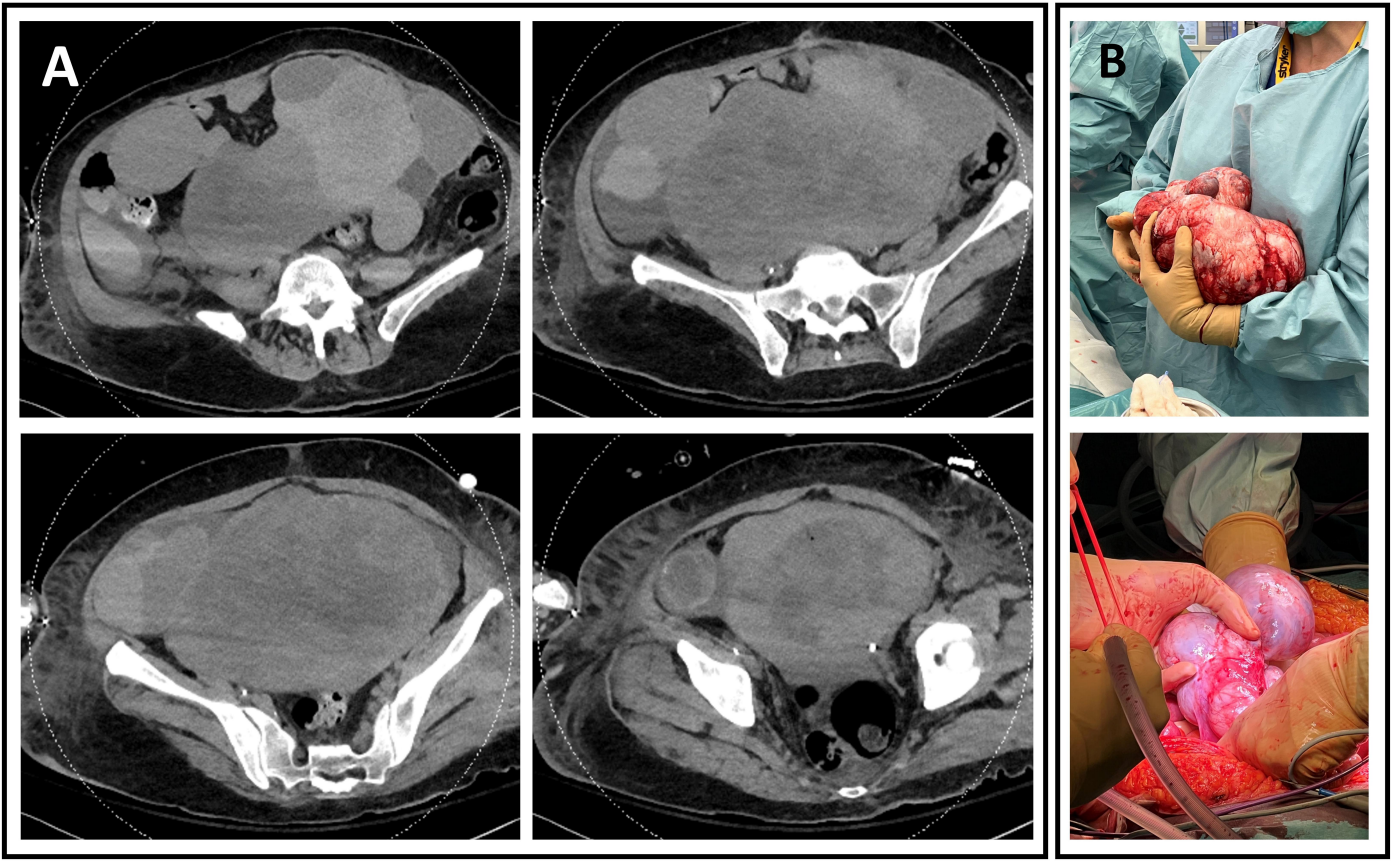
(A) Abdominal CT scan showing a large, heterogeneous, fleshy, and hypervascularized cystic mass measuring 27.2 cm wide, 14.1 cm deep, and 21.7 cm high. (B) Perioperative excision of two large multiloculated cystic masses. (Top) Left mass, weighing 2,932 g, measuring 27 cm × 20 cm × 20 cm. (Bottom) Right mass, weighing 436 g and measuring 15 cm × 8 cm × 5 cm.
